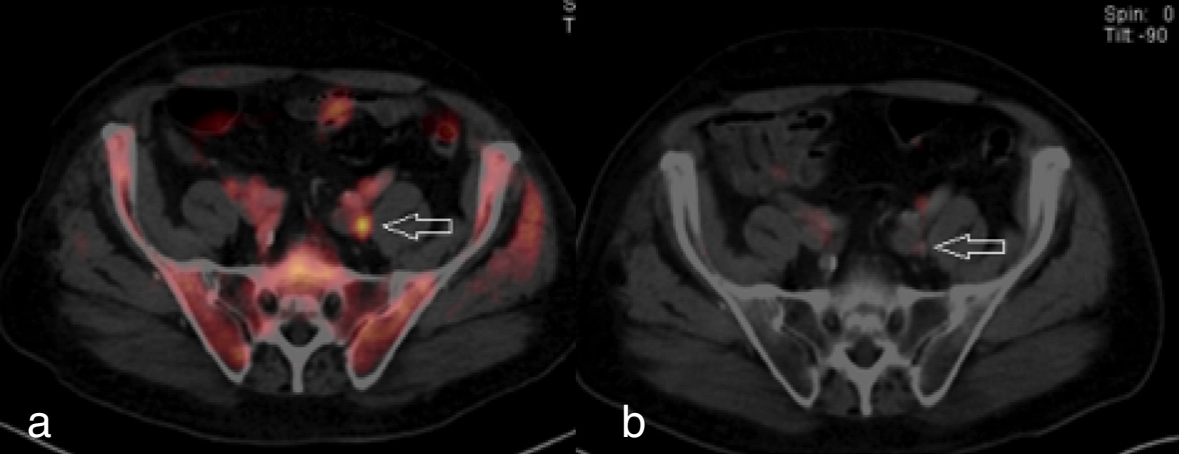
Down-staging by ^68^Ga-PSMA-PET. Choline-PET-CT (a) with elevated tracer uptake of left iliac lymph node. ^68^Ga-PSMA-PET (b) does not confirm the questionable involvement seen by Choline-PET-CT
